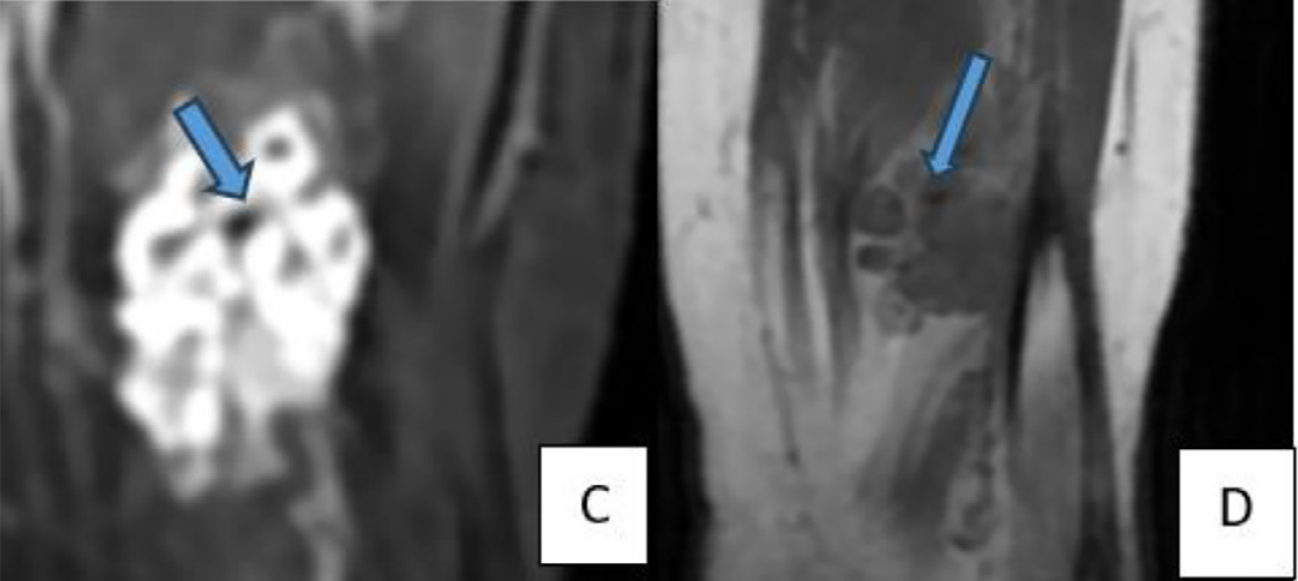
(C) Coronal STIR and (D) coronal T1-weighted sequences showing areas of internal calcification, appearing as signal voids, along with regions of T1- and T2-hypointensity.
